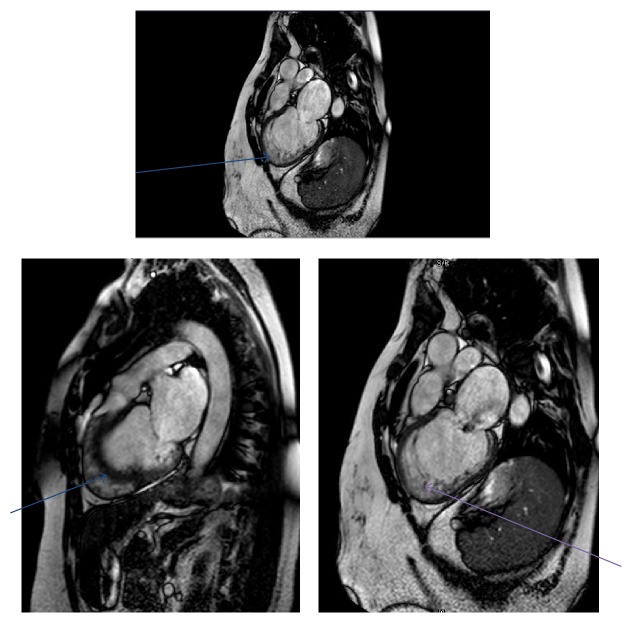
Cine MRI, morphology, phase-contrast, and contrast cardiac MRI.
